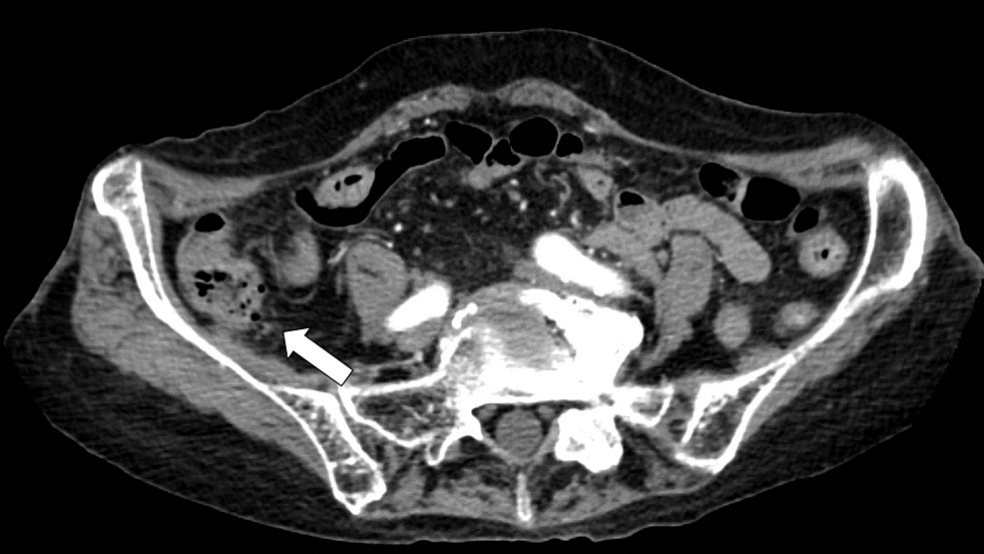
Enhanced computed tomography showing multiple diverticula with extra-luminal gas in the ascending colon
